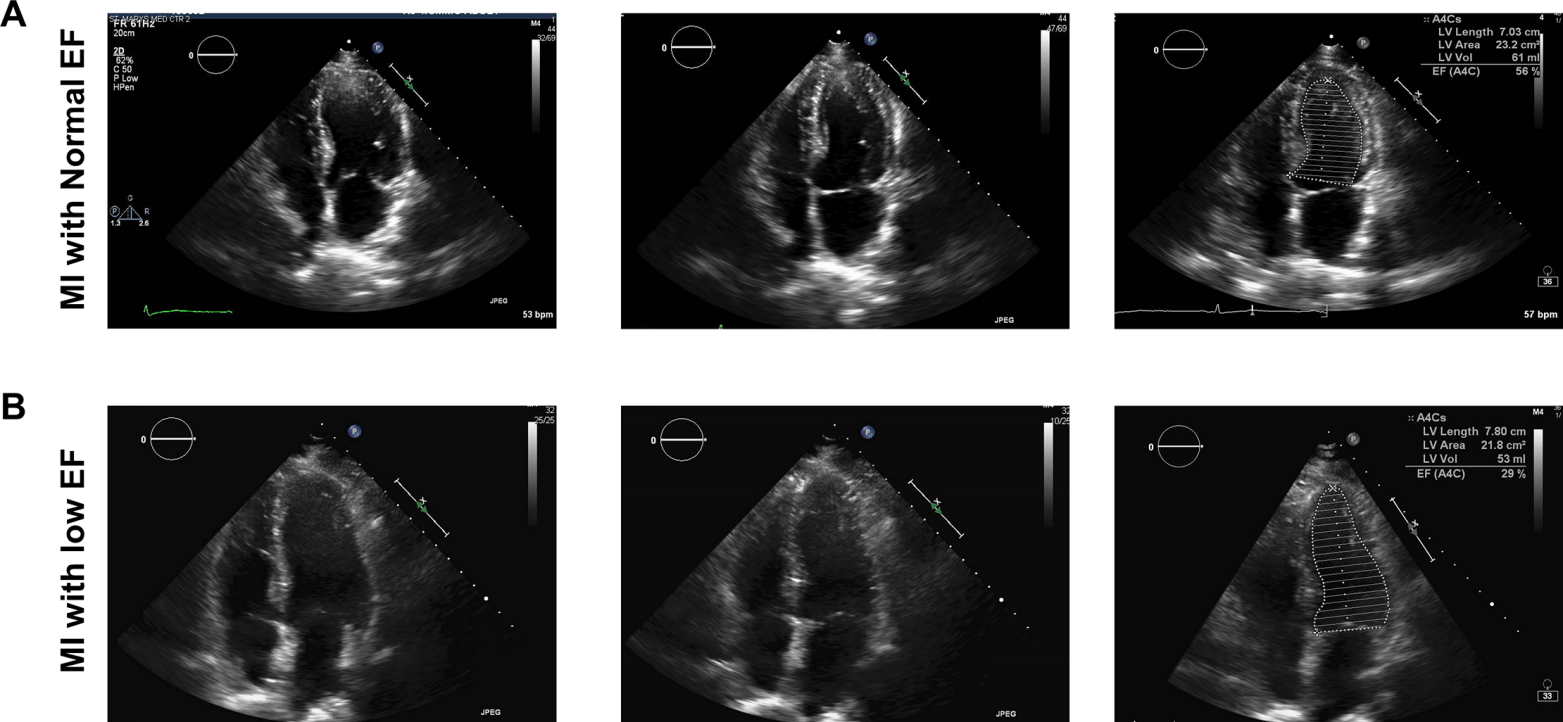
Representative transthoracic echocardiographic images from post-MI patients performed within 48 hours of incidence of MI, to evaluate EF. Images were assessed using biplane method in end diastole and end systole respectively. (A) Evaluation of cardiac function in patients with (A) MI with normal EF and (B) MI with low EF.
